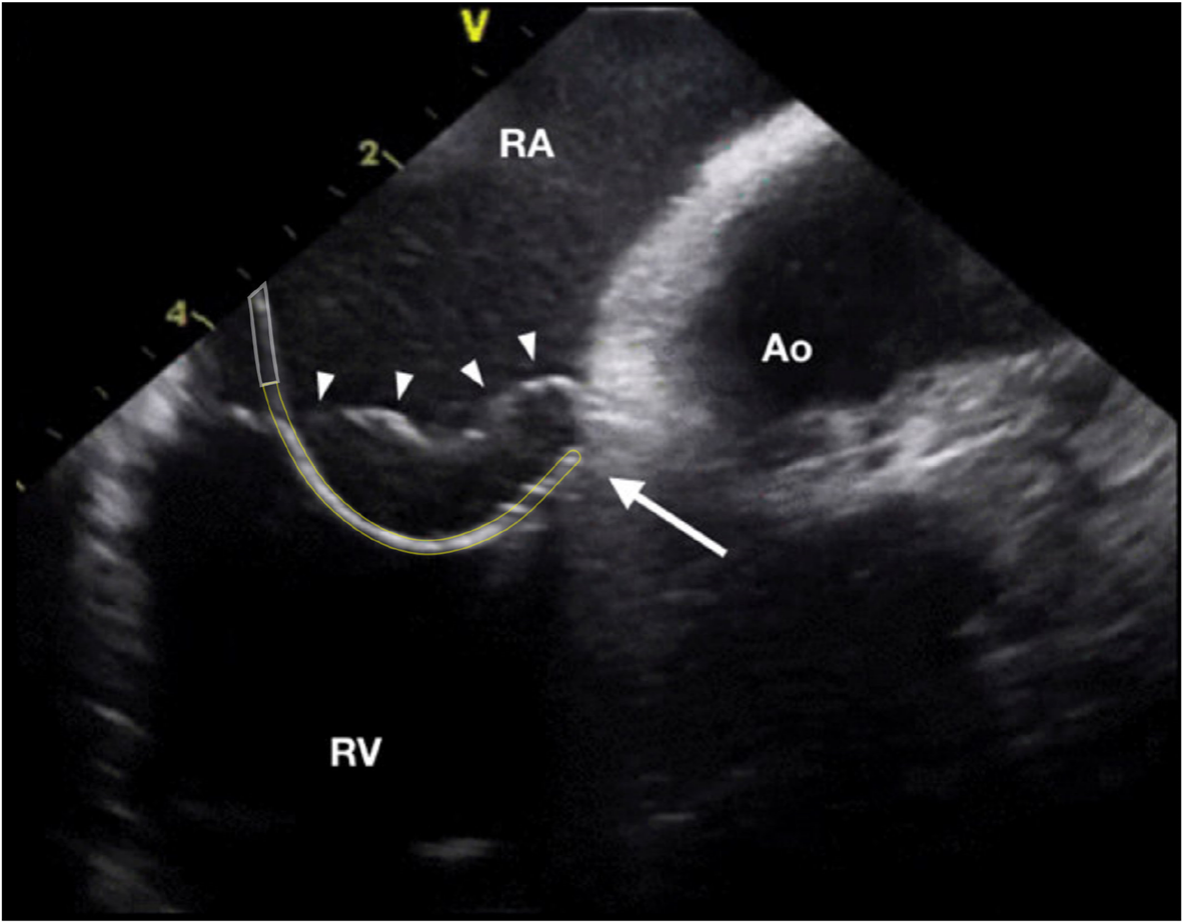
Intra-cardiac echocardiography (ICE) image taken during mapping and ablation of the an-terior right free wall accessory pathway (AP). The 10 Fr (French scale) ICE probe was in-serted into the right atrium via the femoral vein. The ablation catheter was inserted into the right ventricle via a steerable long sheath and the described “loop” manoeuvre was used. White lines mark the presumed location of the steerable long sheath, yellow lines mark the course of the ablation catheter loop. The tip of the ablation catheter is marked with a solid white arrow, the tricuspid valve is marked with solid white arrowheads. RA right atrium, RV right ventricle, Ao aorta.
